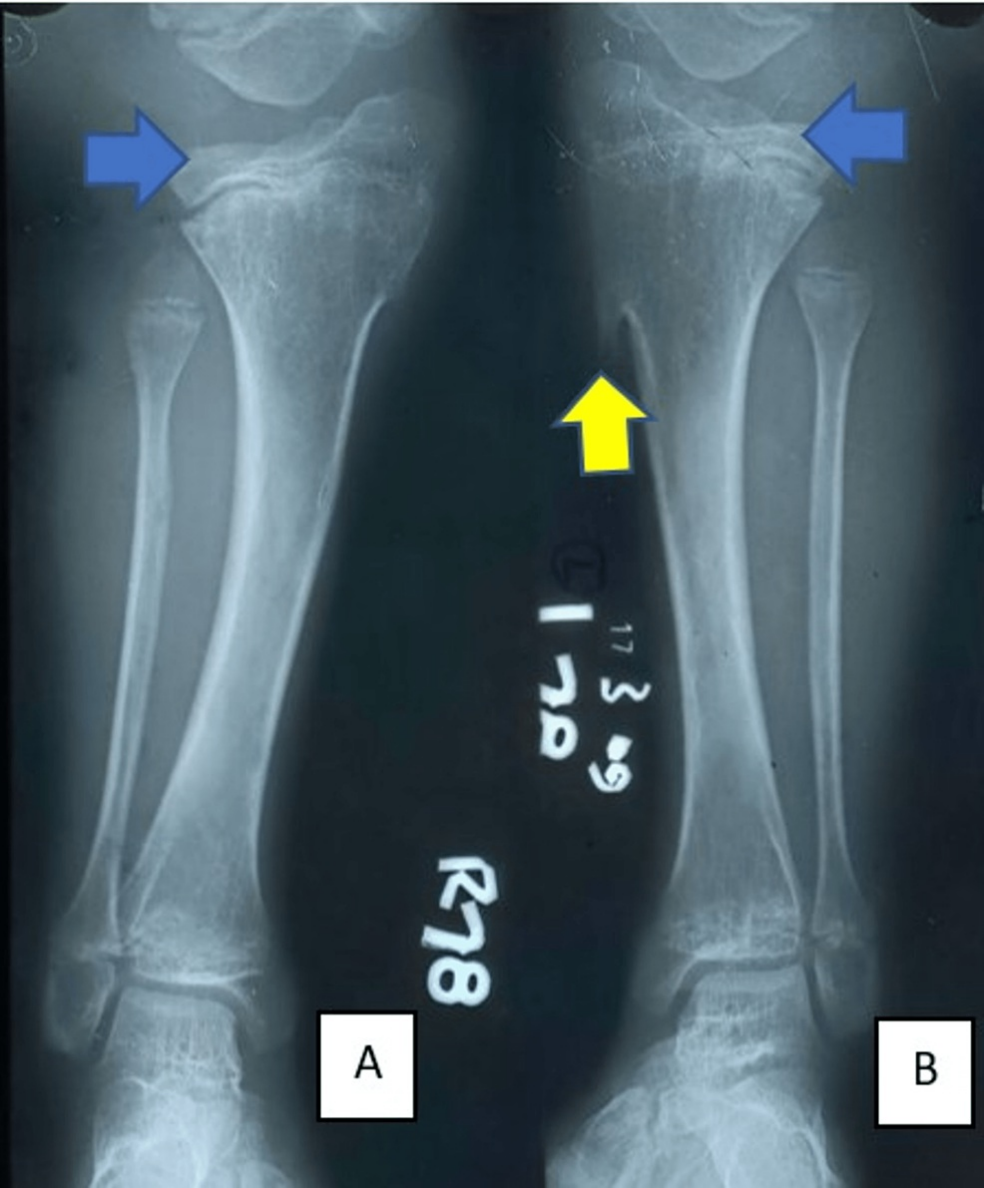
X-ray of bilateral knee with leg: A) dysplastic lateral tibial plateau of right knee (blue arrow). B) Dysplastic lateral tibial plateau of left knee (blue arrow) with exostosis in the proximal tibial region (yellow arrow).
